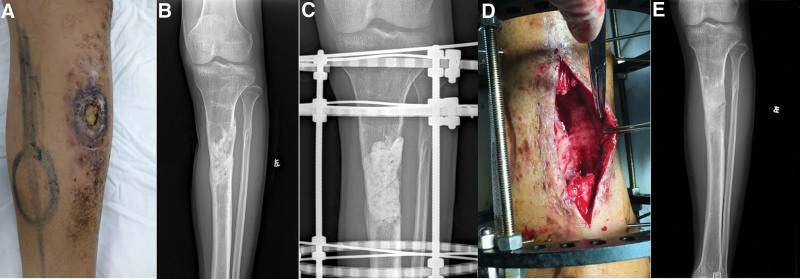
Case 2. A 22-yr-old male suffered from osteomyelitis of the left proximal tibia. (A) Preoperative photograph. (B) Preoperative radiograph. (C) After debridement, the defect was filled with antibiotic-loaded cement spacer and an external ring fixator was applied. (D) Removal of cement spacer and the retention of the induced membrane. (E) A radiograph at 2 yr follow-up showing the bone union.
